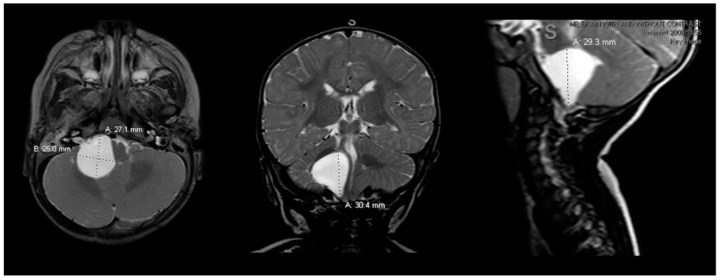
Preoperative brain MRI scan. The preoperative brain MRI scan (left = axial; middle = coronal; right = sagittal) T2 sequences showed a large 2.5 × 2.7 × 3.0 cm homogeneous, hyperintense right cerebellopontine angle cystic lesion causing mass effect on the right pons and medulla. T1 sequences (not shown) were hypointense for the cyst, without contrast enhancement on gadolinium scans. MRI = magnetic resonance imaging.
